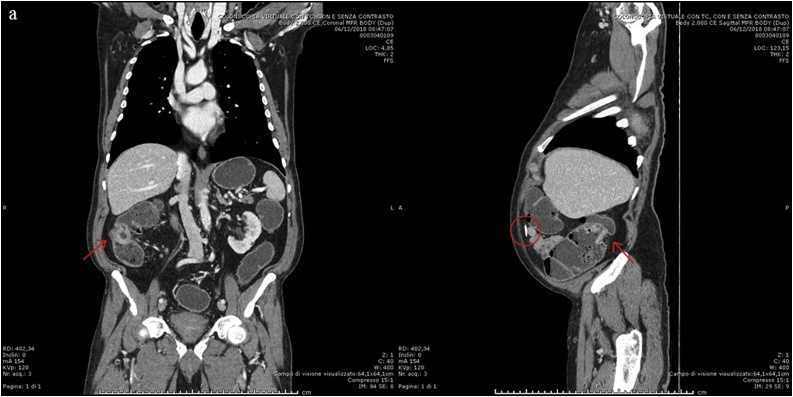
CT Scan showing the tumor of the right colon (arrows). a) Coronal MPR image; b) Sagittal MPR image, in which It is noticeable the VPS Catheter (circle).
